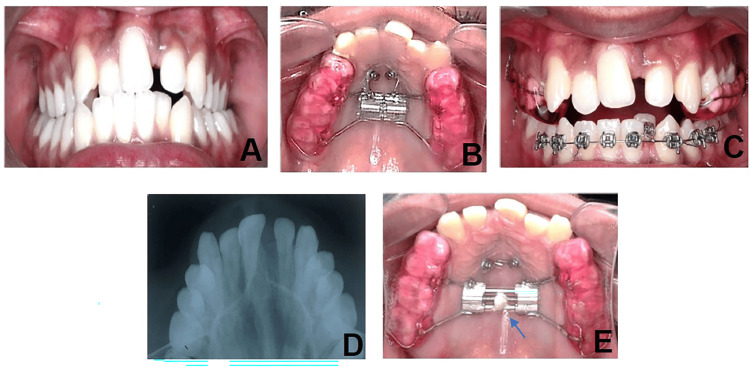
Case 2: Post-expansion appliance arm breakage. (A) Clinical presentation showing transverse maxillary deficiency, (B) MARPE appliance with two miniscrews, (C) clinical confirmation of expansion, (D) radiographic confirmation of expansion, (E) post-expansion fracture of the appliance arm. MARPE: miniscrew-assisted rapid palatal expansion
